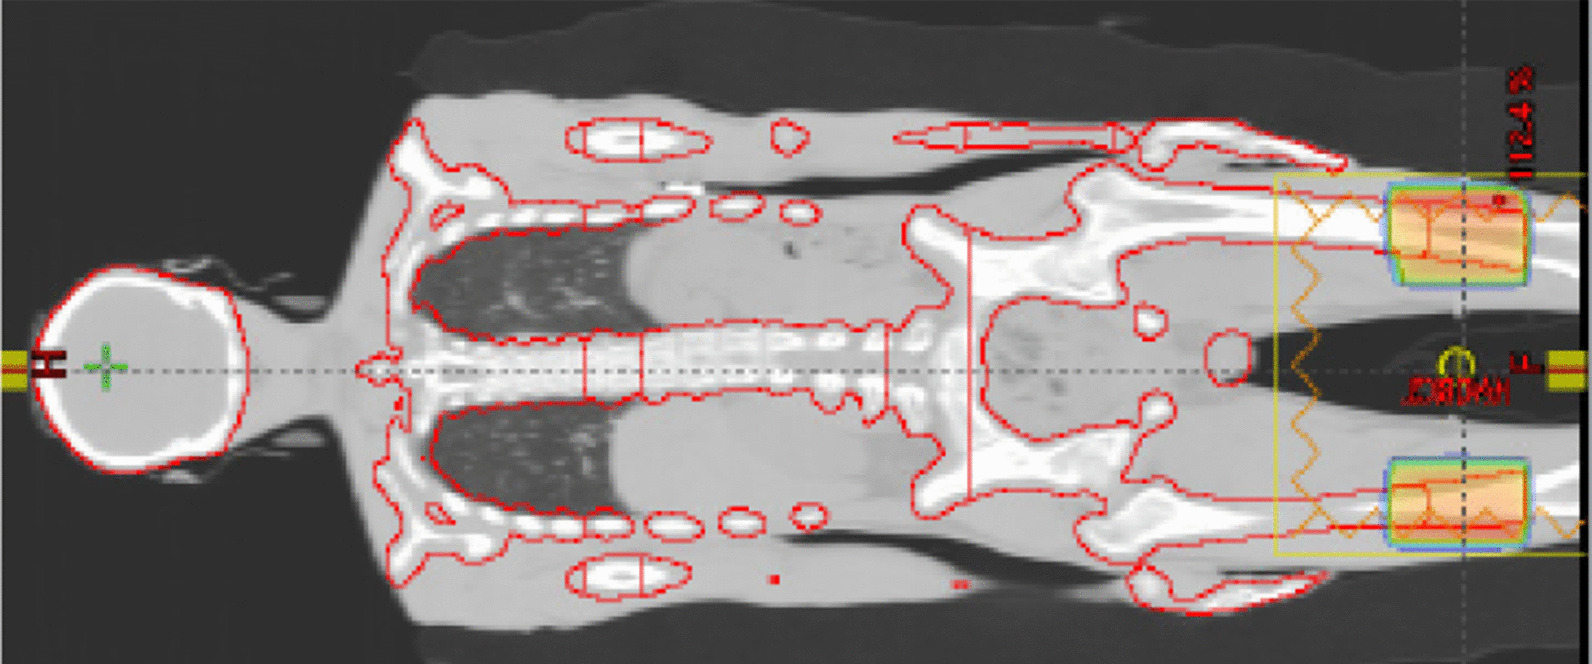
Target volume and junctional plan at thigh level for HFS CT
